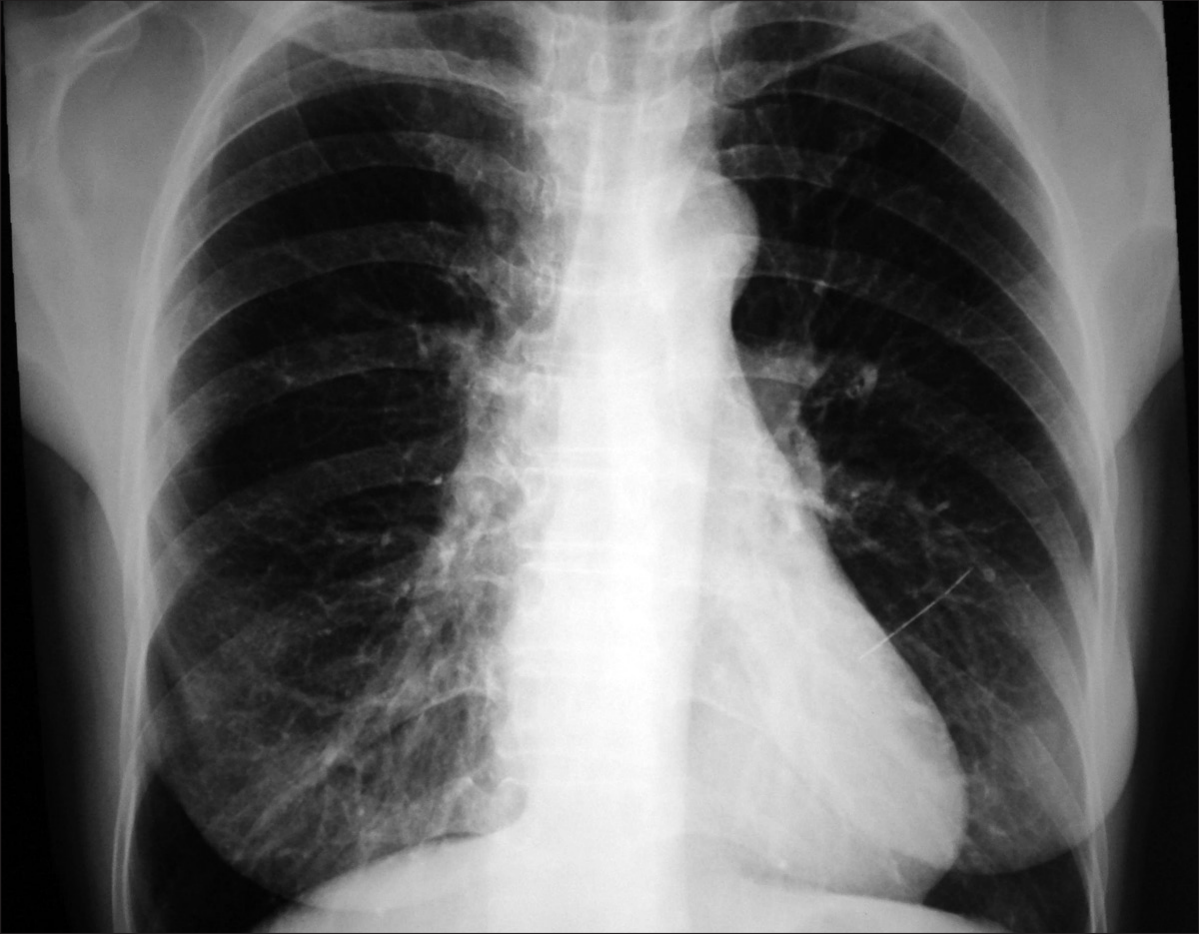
Chest X-ray showing sewing needle perpendicular to the left heart border
